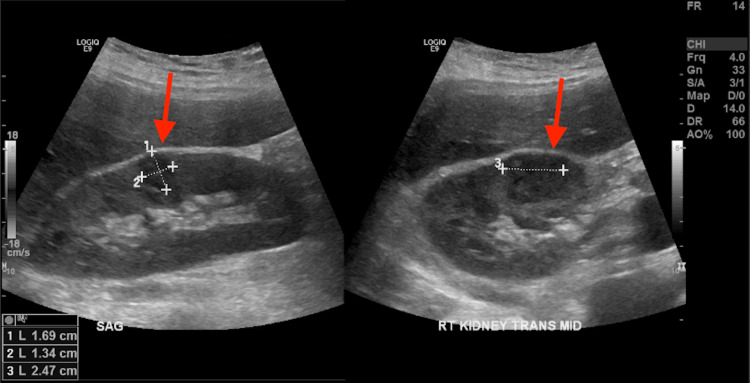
Right upper quadrant ultrasound Arrows delineate slight parenchymal heterogeneity in the upper and mid right kidney, consistent with pyelonephritis.
